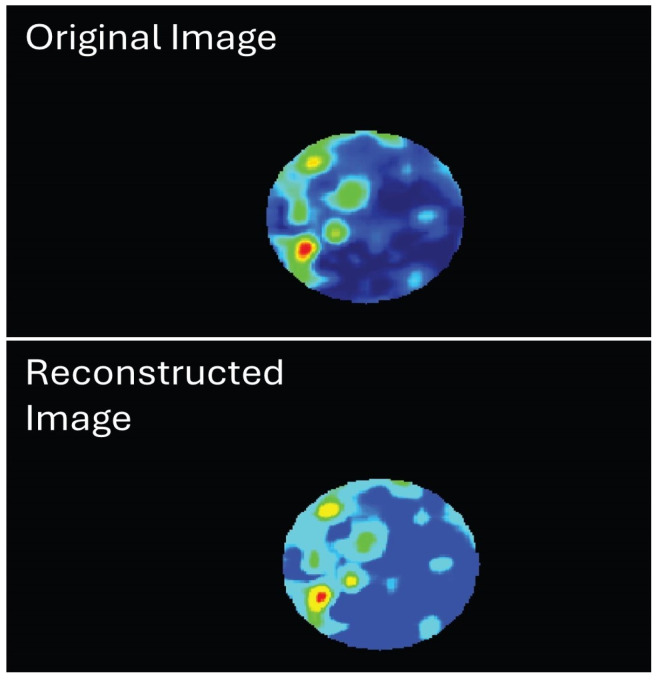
Original ROI image and reconstructed ROI image from strain elastography ultrasound.
